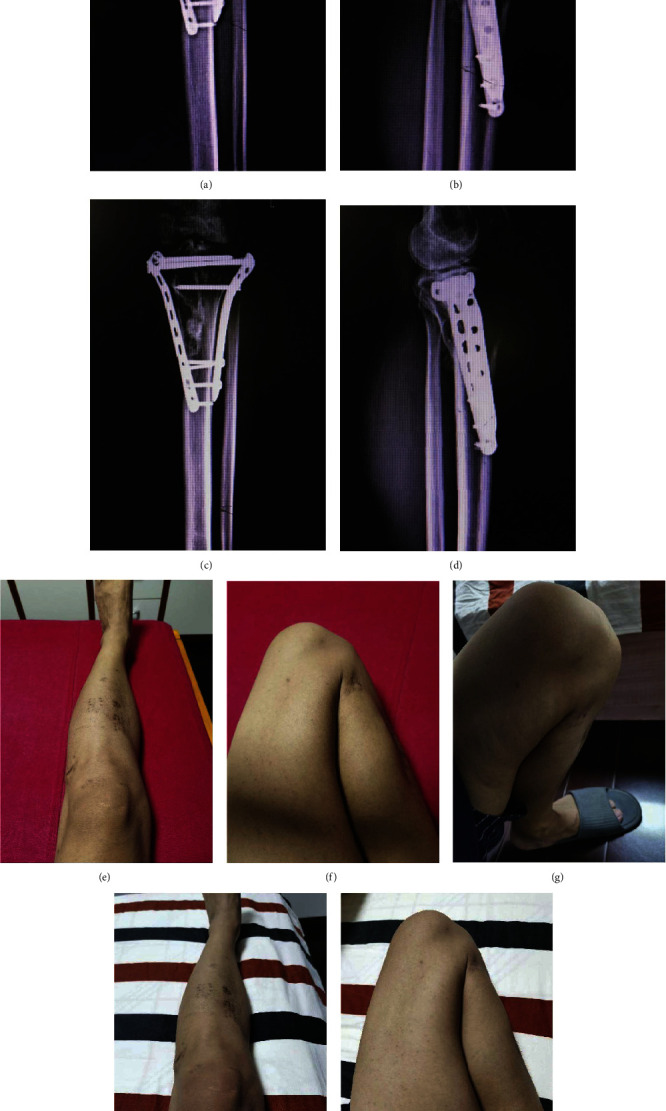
Follow-up results of the 43-year-old male mentioned in Figure 1 were obtained at 6 and 13 months after operation. (a, b) X-rays of tibial plateau obtained 6 months after operation. (c, d) X-rays of tibial plateau obtained 13 months after operation. (e, f) Photographs of the knee joint in extended and flexed positions 6 months after operation. (g–i) Photographs of the knee joint in extended, flexed, and feather-weightbearing positions 12 months after operation.
